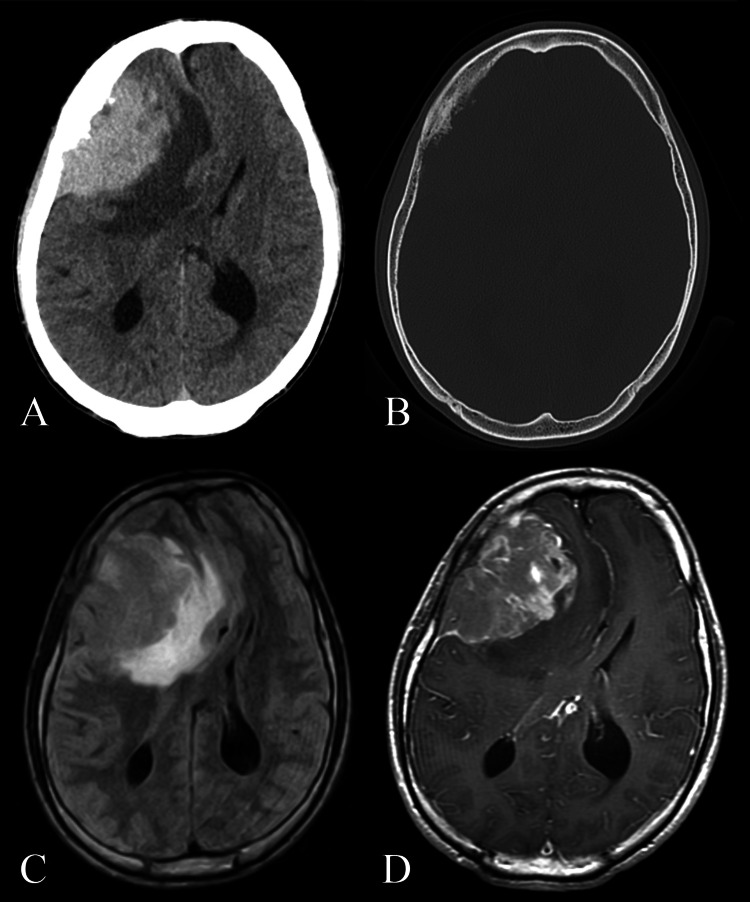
Preoperative images (A) Initial non-contrast head CT scan. (B) Bone window CT image. (C) FLAIR MRI. (D) Gadolinium-enhanced T1-weighted MRI. CT: computed tomography; MRI: magnetic resonance imaging; FLAIR: fluid-attenuated inversion recovery
